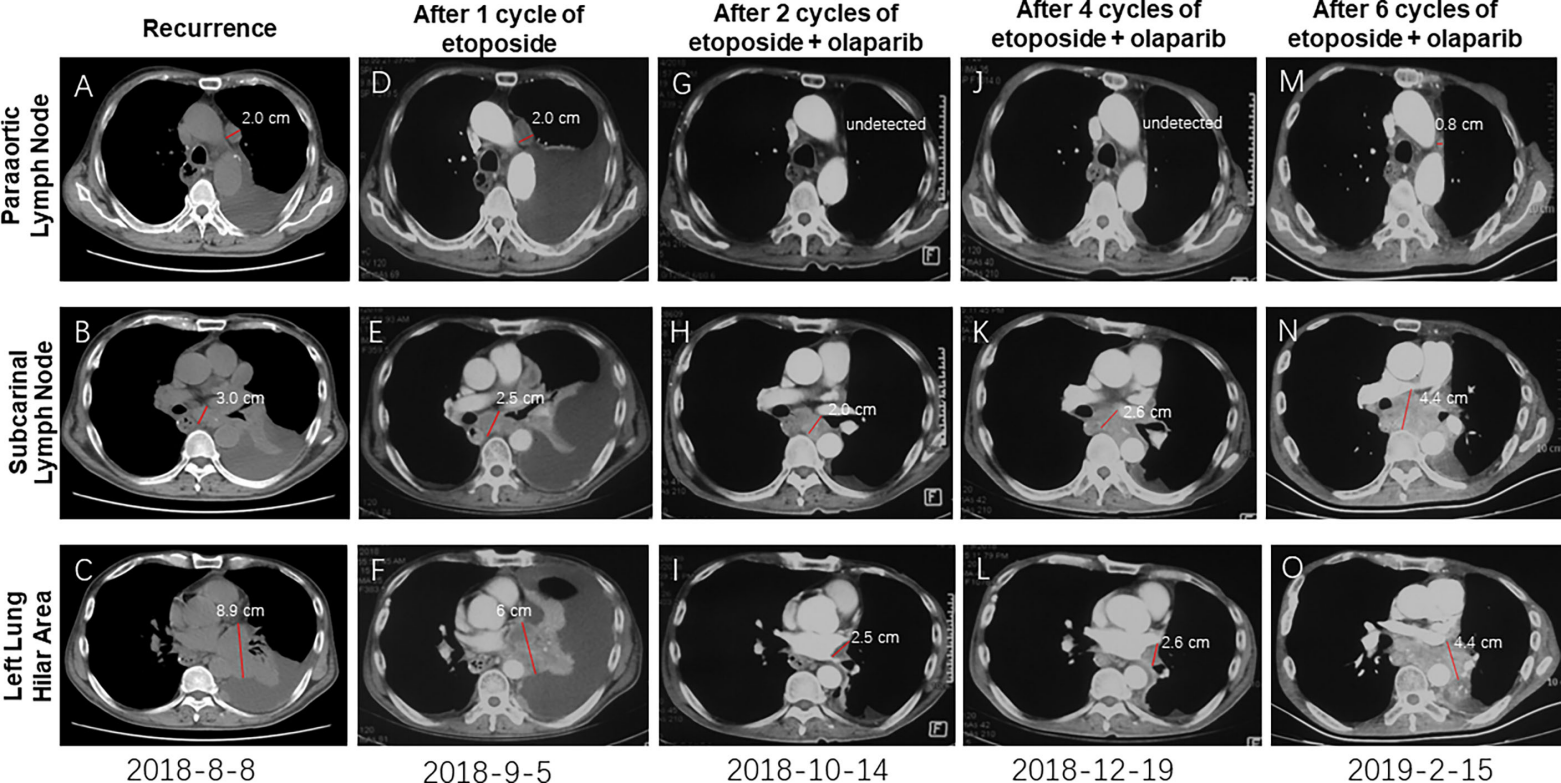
CT imaging showing the recurrent masses and the change of these masses after therapy. Masses after recurrence (A–C); The recurrent masses kept stable after 1 cycle of etoposide (D–F); The recurrent masses were obviously shrunk after 2 cycles of etoposide-olaparib (G–I); These masses kept stable after 4 cycles (J–L) and 6 cycles (M–O) of etoposide-olaparib.
